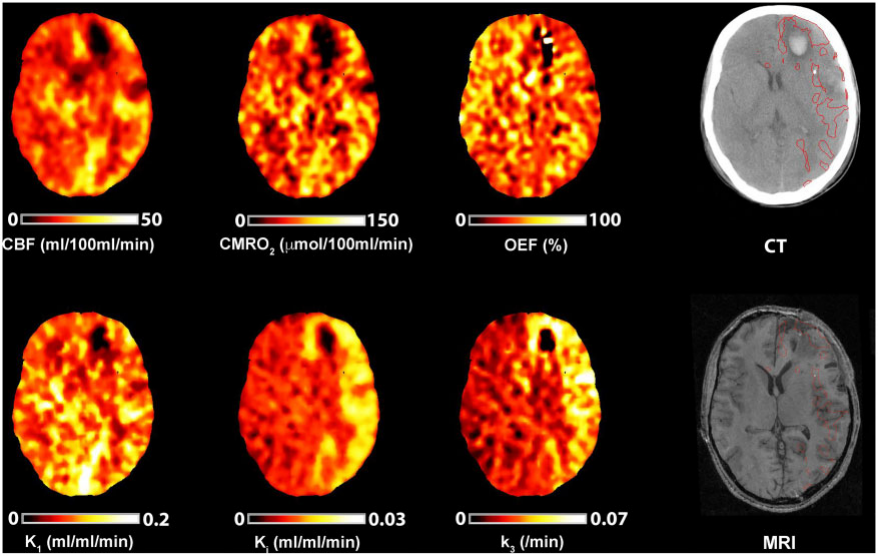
Association between early metabolic derangements and tissue fate. Imaging obtained in a 17-year-old male with moderate TBI following a road traffic accident with initial GCS of 12, but who required sedation and ventilation for control of raised ICP. Co-registered CBF, CMRO_2_, OEF, ^18^F-FDG kinetic parameters and CT imaging obtained on Day 8 following TBI. Plasma glucose during imaging was 5.4 mmol/l. The CT demonstrates left frontal and temporal haemorrhagic contusions and is shown with the T_1_-weighted MRI obtained at 9 months post-injury; the MRI has been non-rigidly registered to the CT. Both structural images are displayed with the region of k_3_ hotspot outlined in red, but both lesion core and penumbra have been excluded from the hotspot to ensure that only increases in k_3_ outside of the lesion identified on CT imaging are shown. The volume of brain within the k_3_ hotspot in this subject was 149 ml and, in comparison with brain that appeared structurally normal, had CBF 19.6 versus 19.6 ml/100 ml/min, CMRO_2_ 55.2 versus 60.2 µmol/100 ml/min, OEF 37.8 versus 42.4%, K_1_ 0.097 versus 0.087 ml/ml/min, K_i_ 0.018 versus 0.012 ml/ml/min and k_3_ 0.058 versus 0.024/min, respectively. The T_1_-weighted MRI demonstrates established lesions within the left frontal and temporal brain regions in close proximity with k_3_ increases outside of lesion core and penumbra identified on the CT image.
